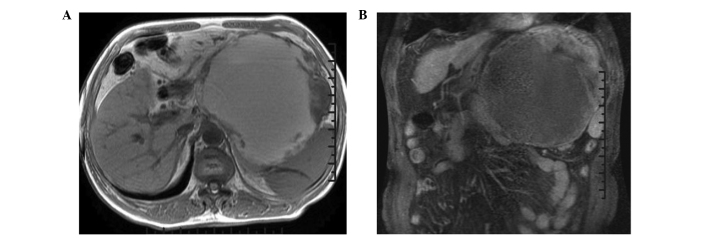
(A) Magnetic resonance imaging revealed a large cystic-solid mass of 17×15×16 cm in size that had grown into the lesser omental bursa. (B) Coronal plane shows the stomach had changed shape due to compression and the mass was closely attached to the gastric wall.
